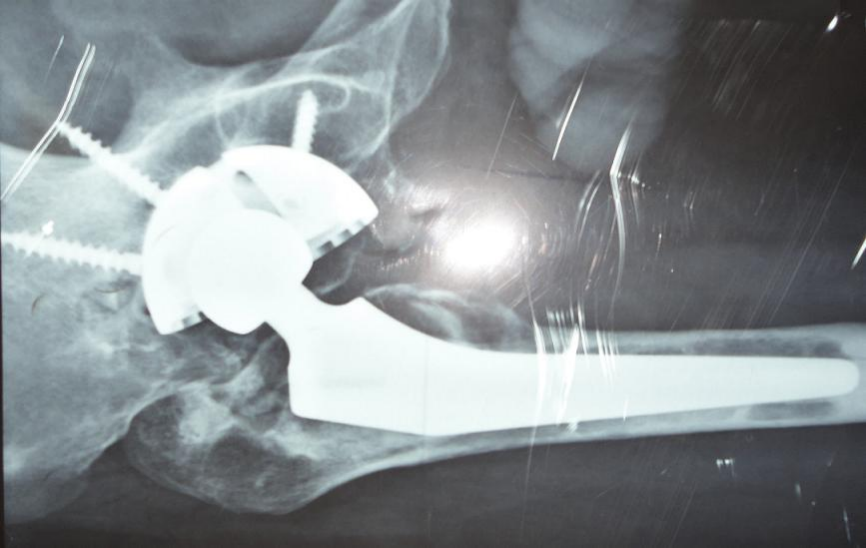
Radiographie standard d'un patient qui présente un descellement aseptique bipolaire
